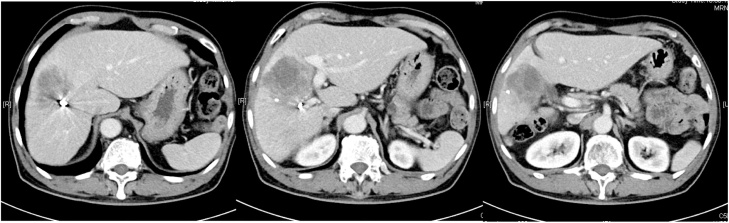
Axial images of CT scan after Y90 therapy: the HCC lesion reduced to 6.5 cm in size.
